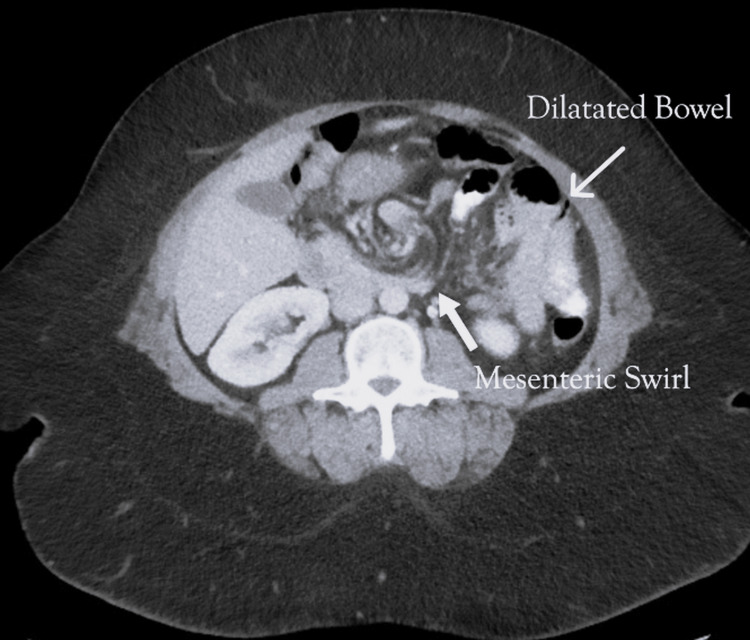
Computed tomography imaging of mesenteric swirling and proximal bowel dilatation.
